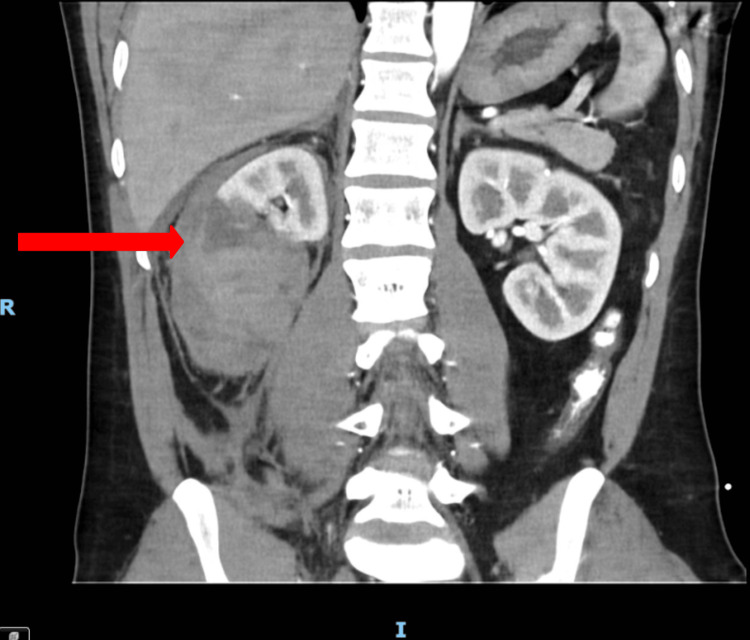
CT kidney triple phase (coronal view) taken on day three of hospital admission The red arrow points to the perinephric hematoma. The letter R depicts the right-hand-side of the patient. The letter I depicts the inferior aspect of the patient.
